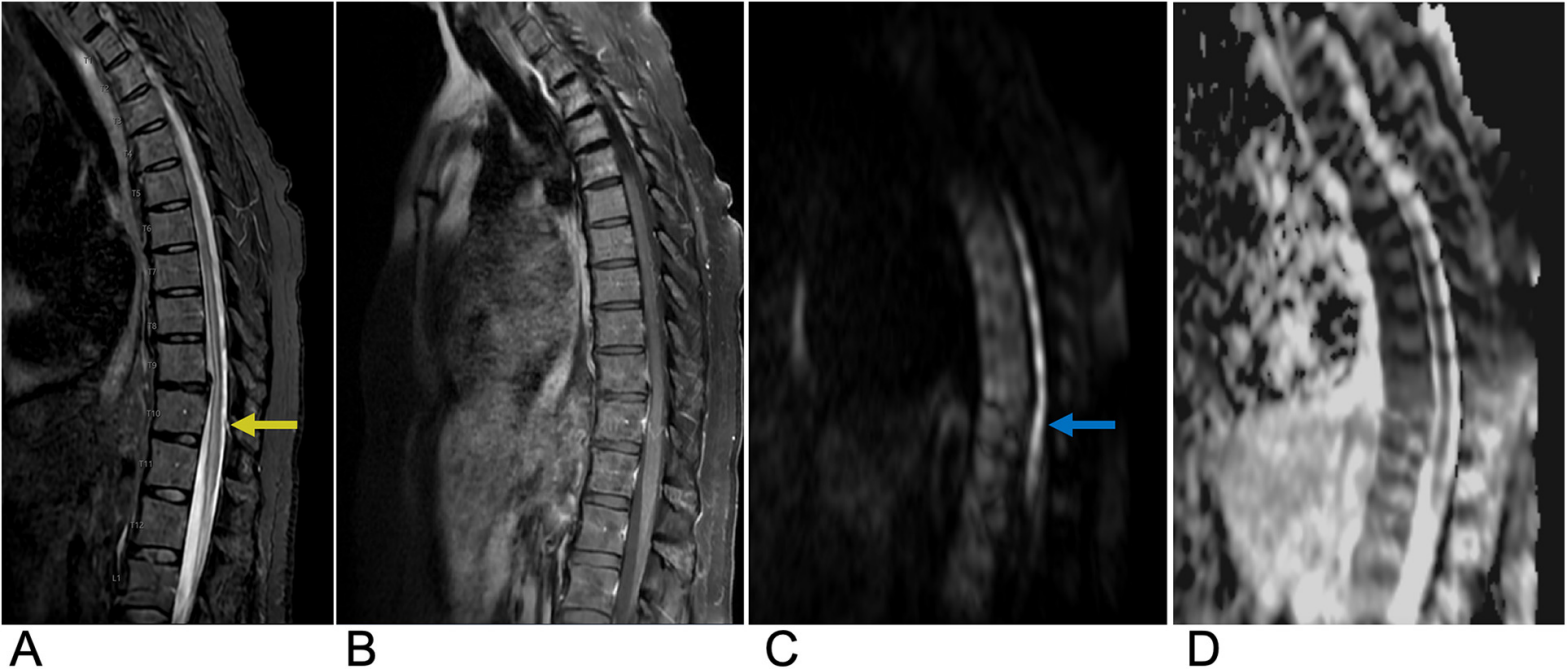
Spinal cord infarction. Sagittal T2 (A), sagittal T1 FAT + C (B), sagittal DWI (C), and sagittal ADC (D) MRI of the thoracic spine demonstrating a long segment of abnormal restricted diffusion (blue arrow) from approximately T5 to the conus medullaris with associated central cord T2 signal abnormality (yellow arrow) extending from T5 through the conus medullaris concerning for spinal cord infarct.
